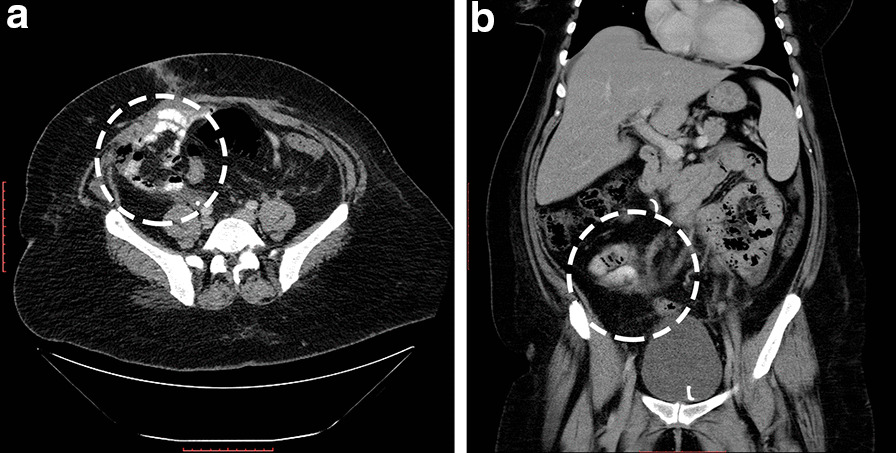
Transverse (a) and coronal (b) computed tomography images showing full neoplastic remission after 18 months of letrozole 2.5 mg per day without any evidence of a primary uterine tumor marked by the dashed circle
